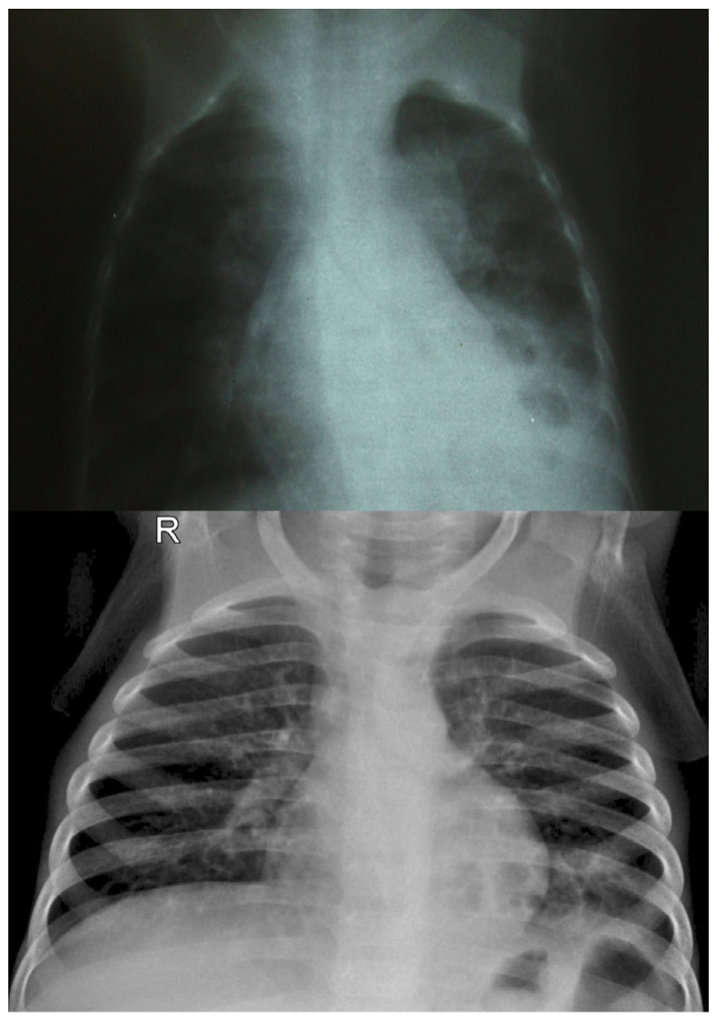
Visual comparison between standard and digital radiology. Note: A typical screen-film X-ray (upper image) in comparison with a digital X-ray (lower image).
